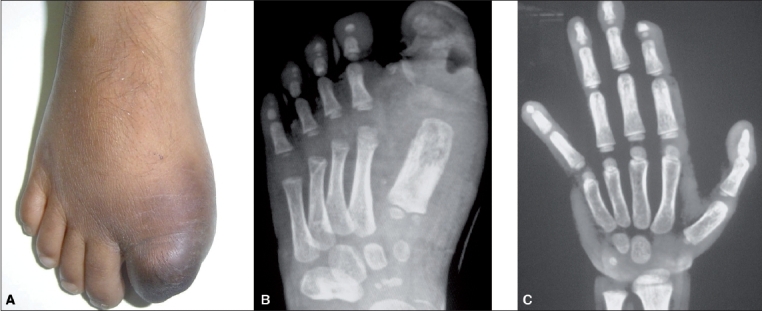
(A) Case 1: Clinical photograph of both hands showing changes of discoloration and dry gangrene of the right middle finger and the swollen finger tips. (B) Case 1: Radiograph of the left foot shows chronic osteomyelitis of the first metatarsal bone, disorganization of the metatarso-phalangeal and inter-phalangeal joints of the great toe along with irregular resorption of the phalanges, soft tissue swelling and ulceration. (C) Case 1: Radiograph of the left hand shows acrolysis of many fingers
